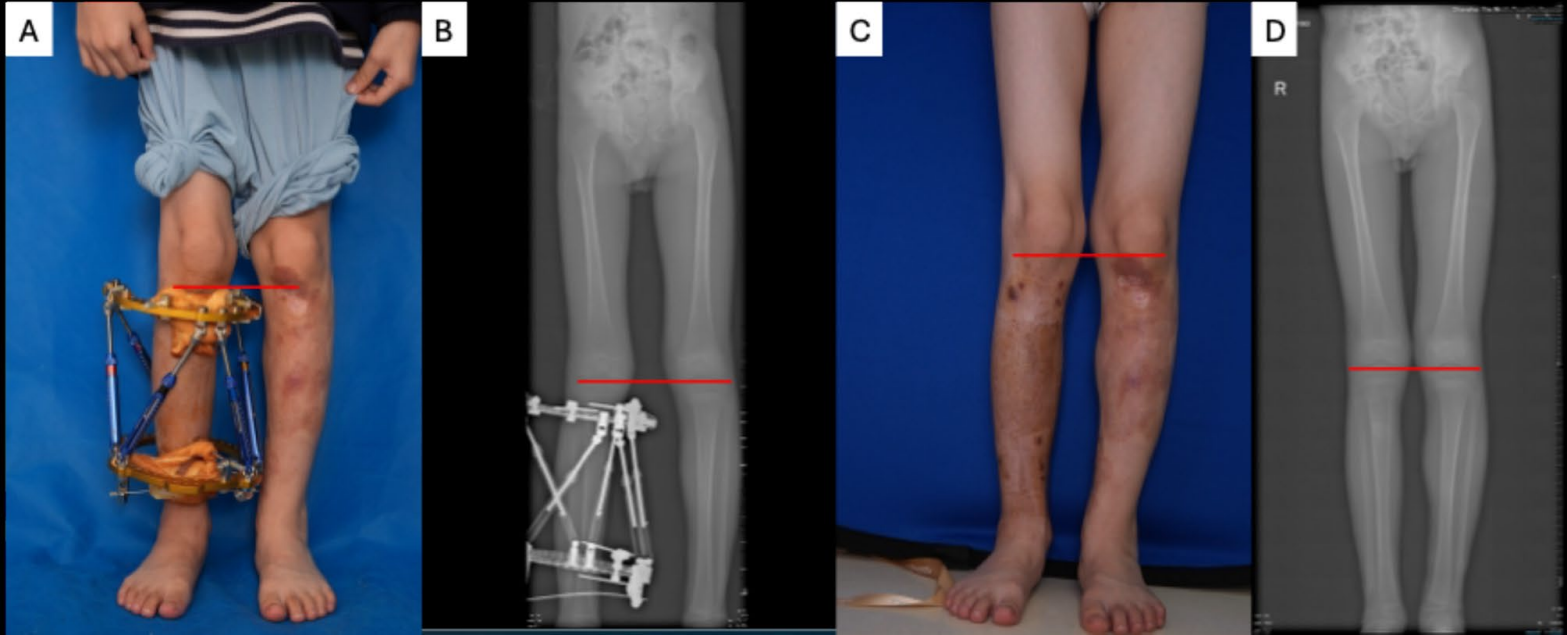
Clinical manifestation and radiograph of the patient outcome. A: The leg length discrepancy was treated by Illizarov Technique where the same knee level. B: Digital radiography of the lower extremities showed same length tibias in lesional and healthy one. C: The same knee level during the patent’s post-operation clinical follow up; D: Same knee level in Digital radiography during the patent’s post-operation clinical follow up
